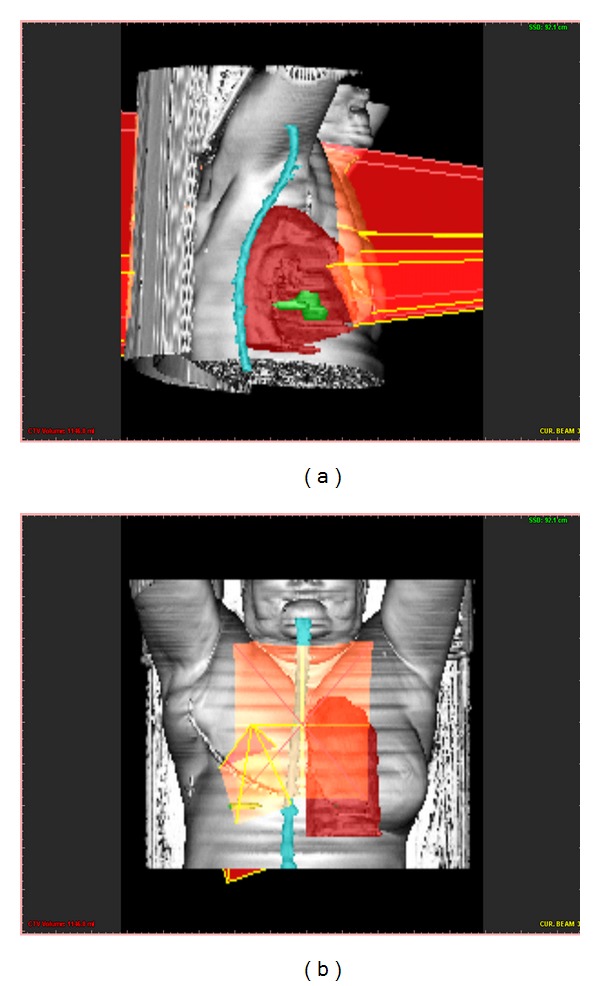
3D beam object reconstructed with patient's CT data. Green object: tumour.
